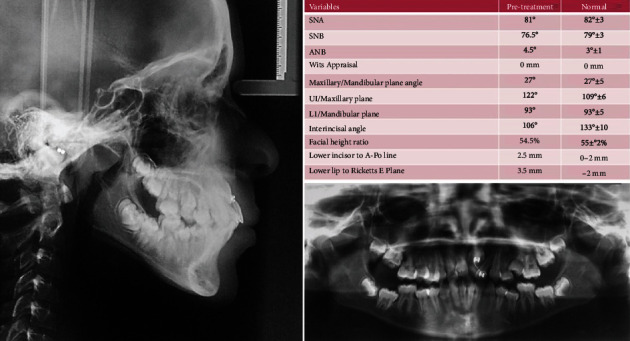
Pretreatment radiographs and analysis.
